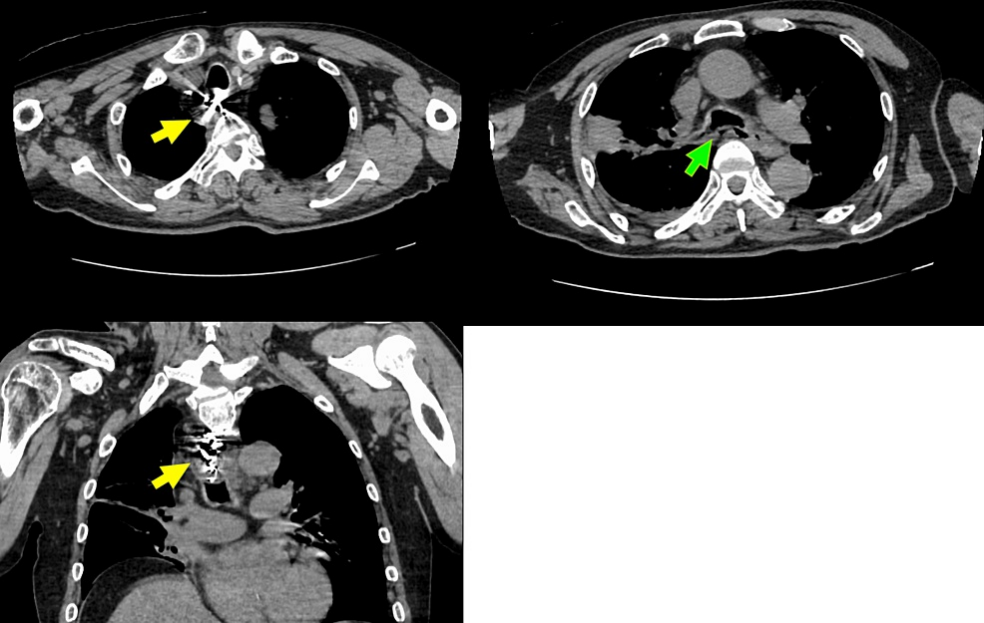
Chest CT findings: presence of a denture within the esophageal lumen and extraluminal air adjacent to the esophagus.
